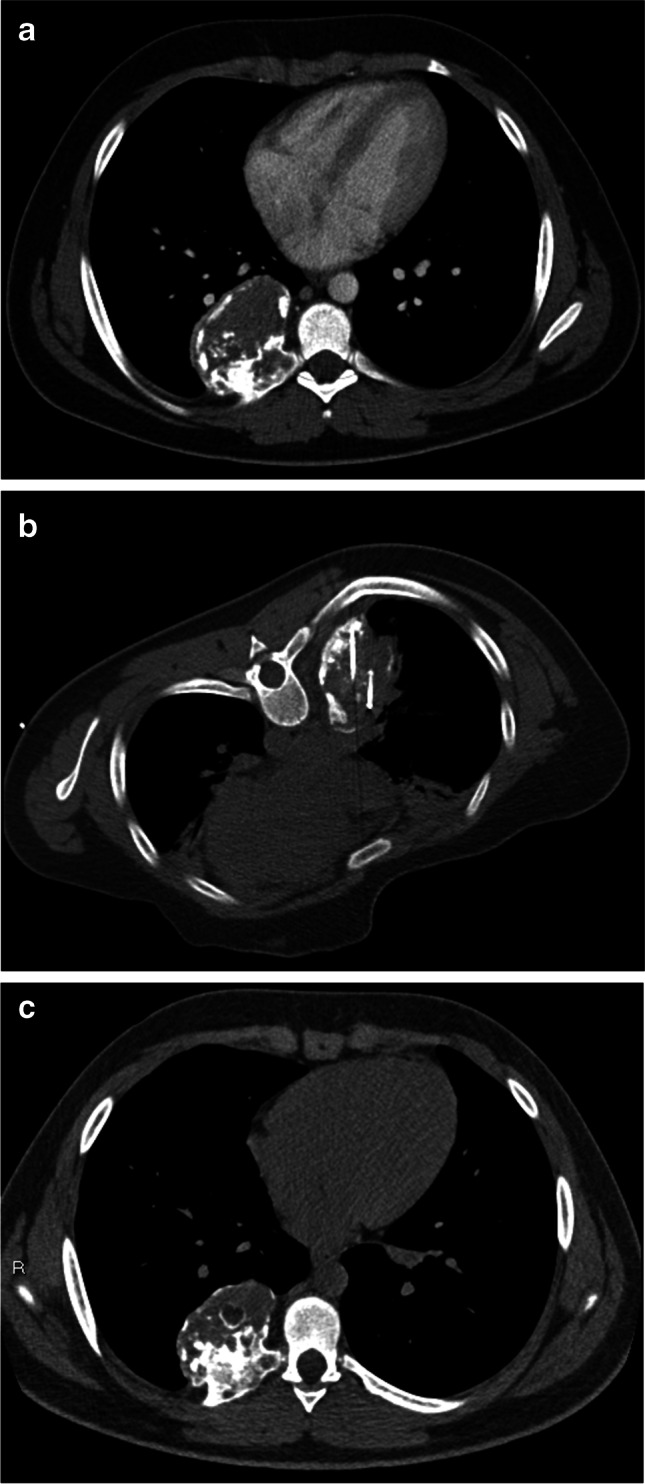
Incidental mesenchymal hamartoma in a 163-month-old boy (patient 4) treated with cryoablation. The mass, involving the 6th–7th–8th right ribs, was found at chest radiography. a Axial chest CT with contrast agent confirms the finding. b, c The boy underwent two sessions of cryoablation (intraoperative axial CT, b) with a slight dimensional increase in the lesion, as shown by the axial CT scan at last 18-month follow-up (c)
